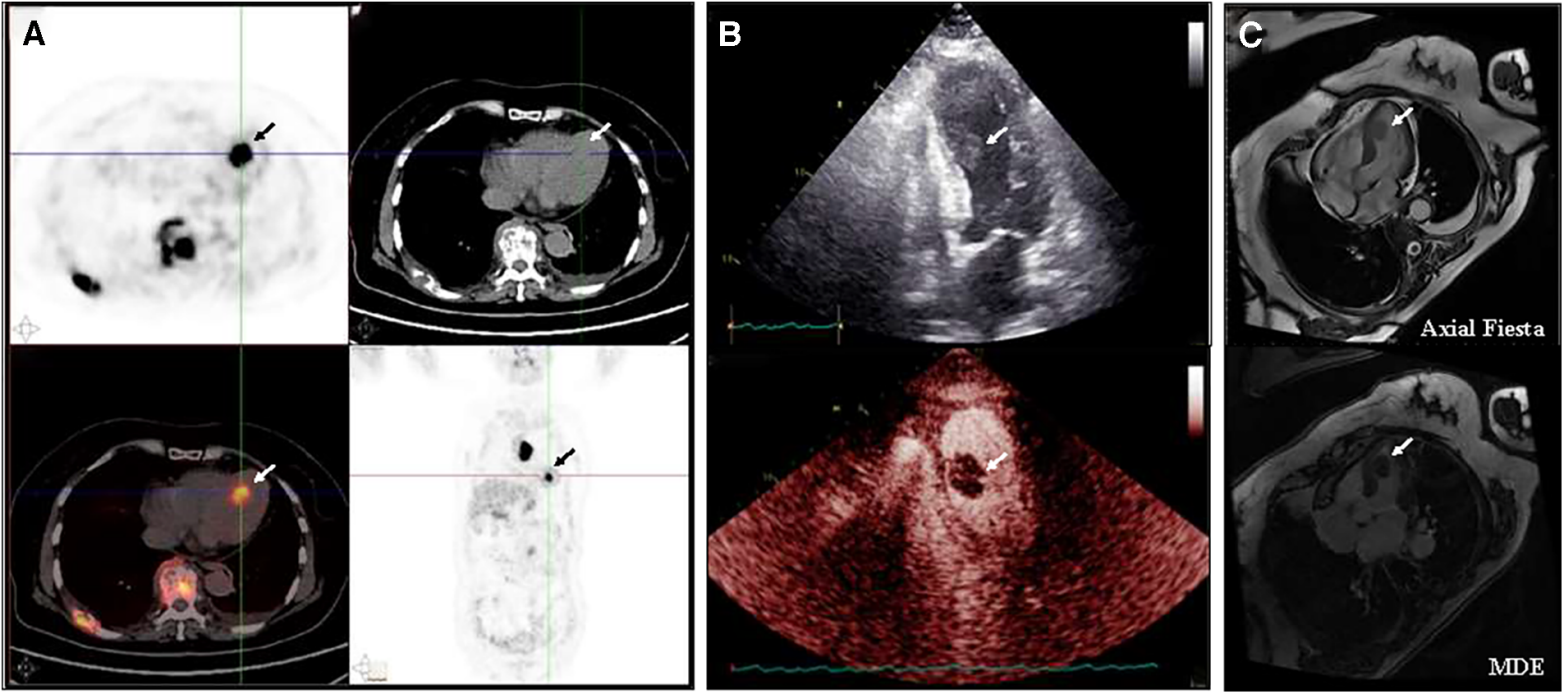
Baseline imaging of the left ventricular mass (A) PET/CT indicated multiple masses in the left ventricle, gallbladder, right adrenal gland, and right vertebral column, along with significant lymph node enlargement and widespread bone destruction, all exhibiting markedly increased FDG uptake. (B) Echocardiography showed an additional echogenicity measuring 14 × 17 mm in the proximal segment of the left ventricular septum, with an irregular shape, tissue-like echogenicity, and activity. A detectable filling defect in the septum near the apex is observed, with an irregular shape, approximately 12 × 22 mm in size, broad at the base, highly mobile at the attachment point, and containing a relatively large amount of contrast filling material. (C) CMR showed a mass measuring 1.6 cm × 1.1 cm × 2.1 cm at the left cardiac chamber septum. Long-axis four-chamber myocardial delayed enhancement (MDE) showed that the left ventricular mass appeared non-gadolinium enhancement at baseline.
